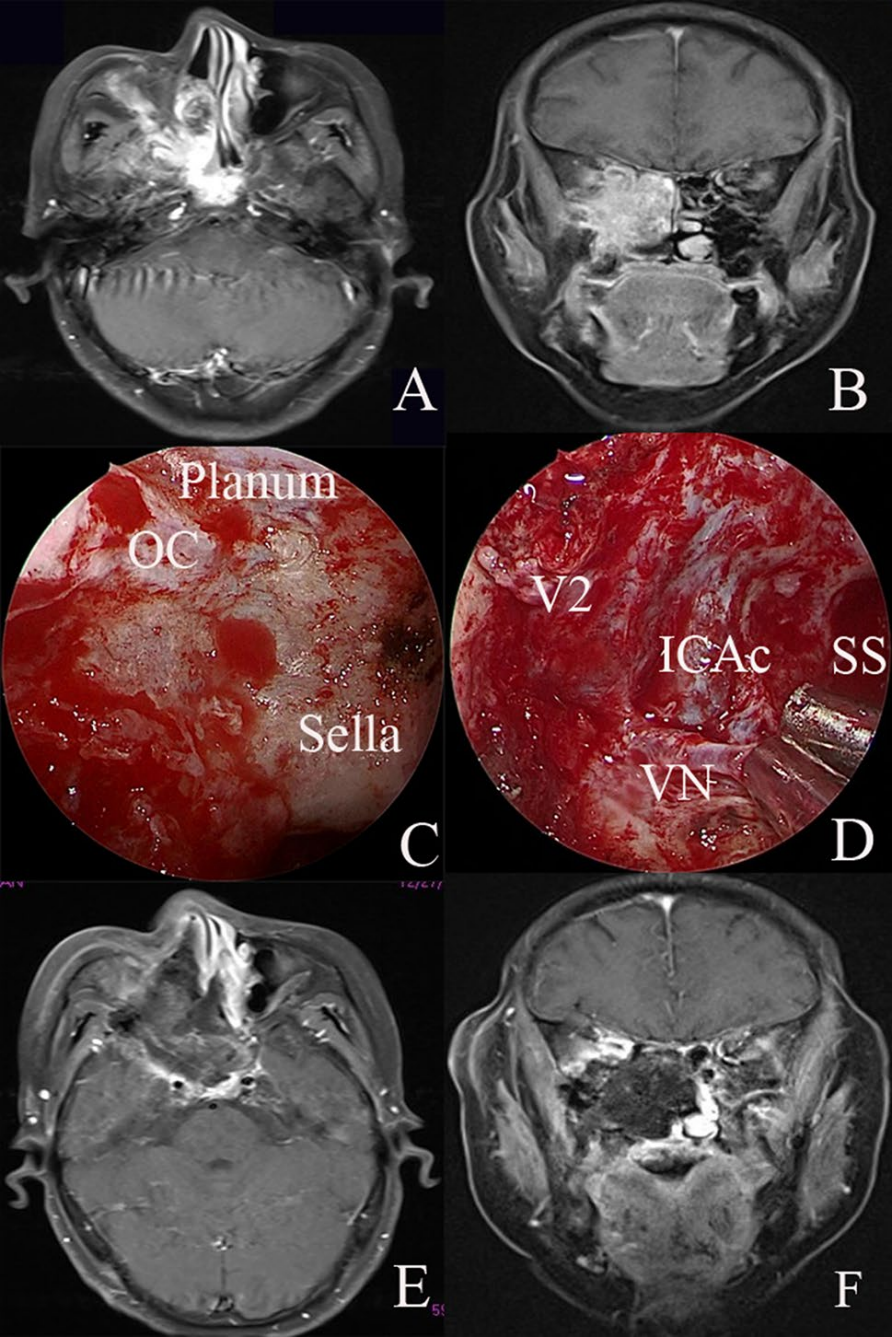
Case 2* Recurrent sinonasal adenoid cystic carcinoma. a, b Preoperative MRI indicated that the tumor involved the infratemporal fossa, upper parapharyngeal space, cavernous sinus and middle skull base. c The sphenoid sinus and lateral wall structures, including the paraclival internal carotid artery and optic nerve, were exposed. d The paraclival internal carotid artery was located. The foramen ovale was located and showed tumor involvement. The maxillary nerve was resected by drilling off the bone, and the floor of the middle cranial fossa was exposed. e, f Postoperative MRI demonstrated complete resection of the tumor. *OC* optic canal, *ICAc* paraclival segment of the internal carotid artery, *VN* vidian nerve, *V2* maxillary nerve, *SS* sphenoid sinus
